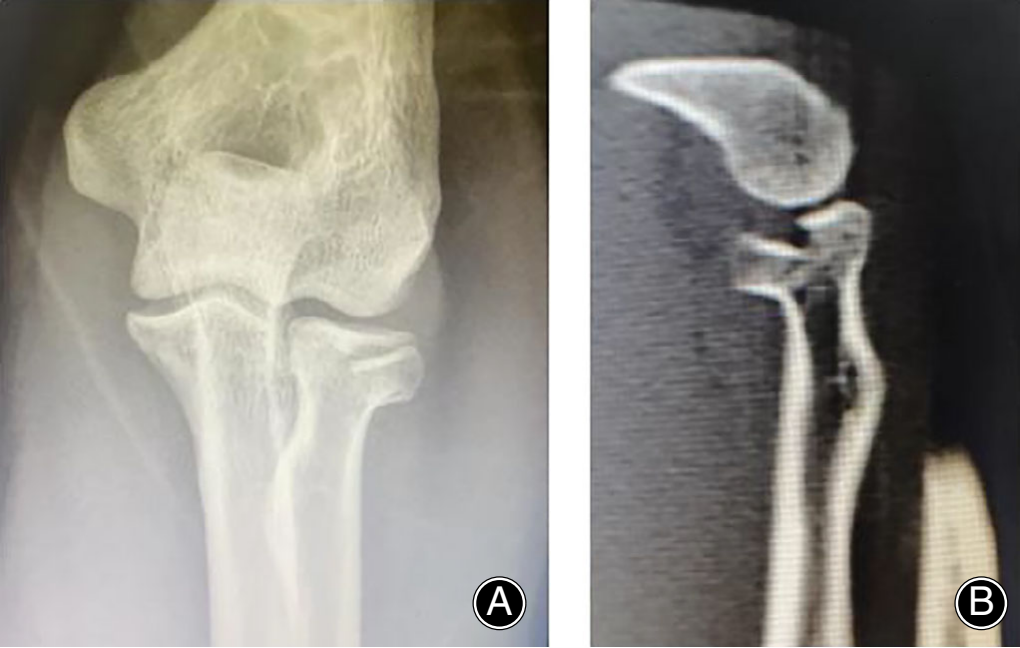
The common characteristics of the Mason type II radial head fractures were obtained by X‐ray and CT images. The CT machine was used to scan the elbow. A total of 380 slices (0.5 mm thickness) tomograms were saved in the dicom format.
